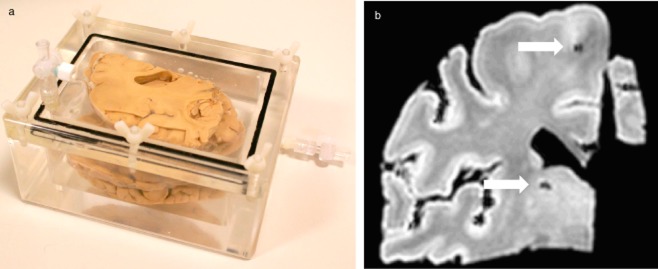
(a) Perspex chamber loaded with formalin fixed frontal lobe brain slices used to present tissue for magnetic resonance imaging (MRI). (b) Susceptibility weighted 3T MRI scan image of a representative slab of brain tissue showing two signal voids (arrows) with the characteristics of microbleeds.
